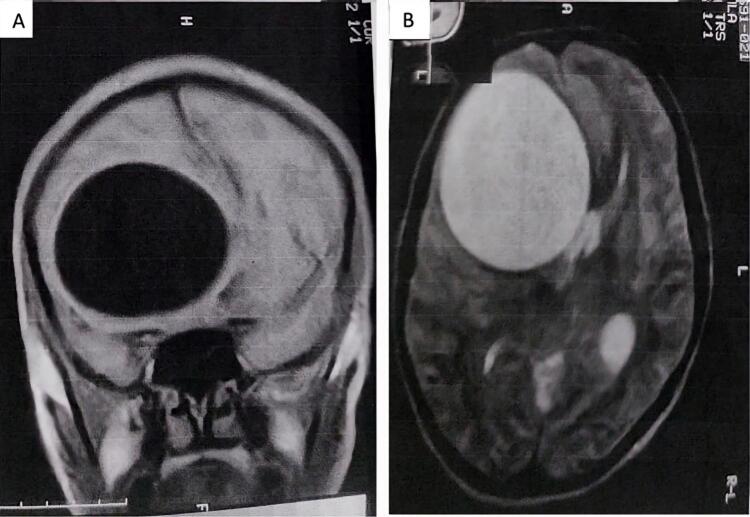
Brain MRI: Rounded well-limited lesion in Hyposignal T1 (A), and Hypersignal T2 (B) with a maximum diameter of 50 mm.
